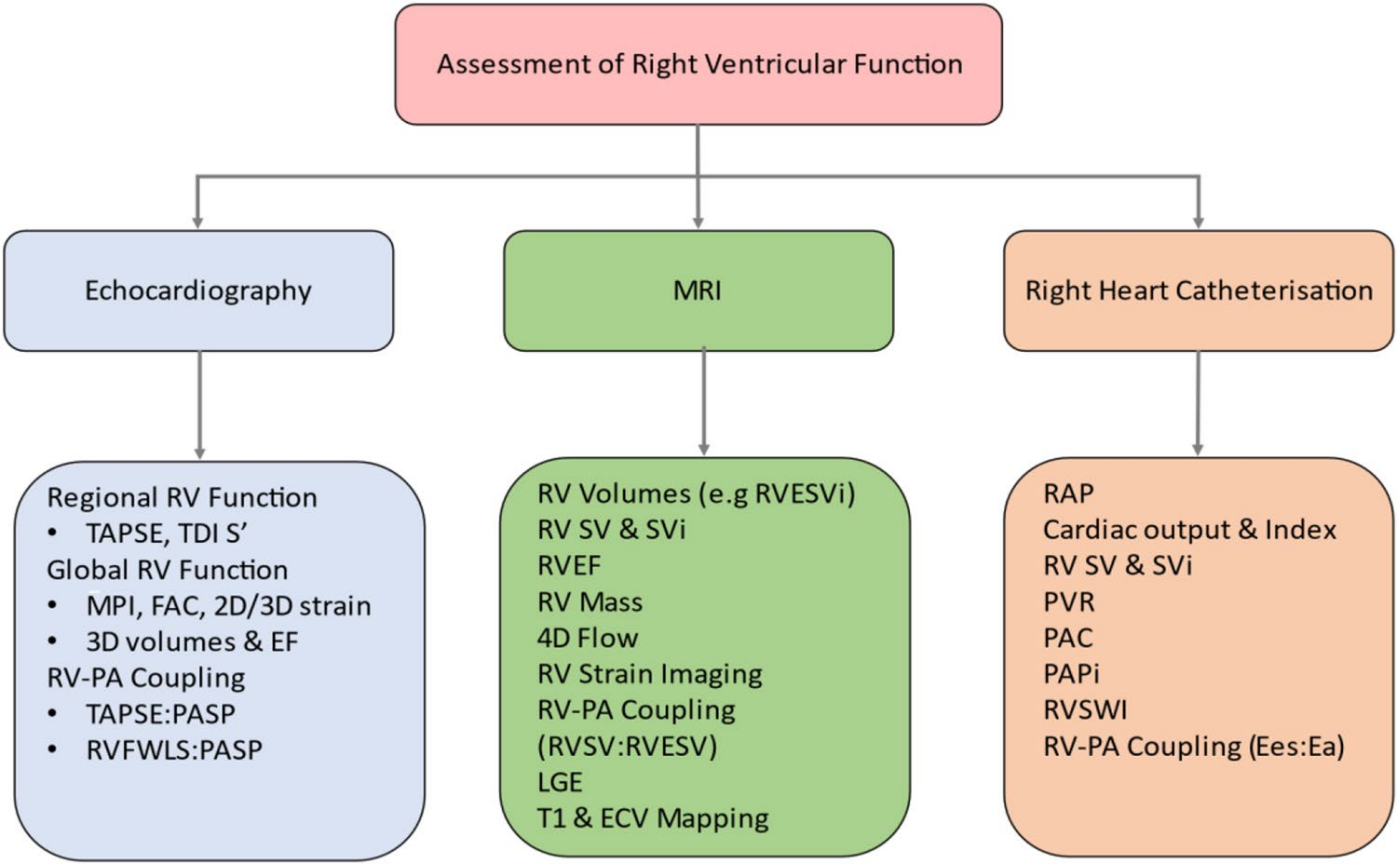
Modalities and parameters used for the assessment of right ventricular function. MRI, magnetic resonance imaging; RV, right ventricular; TAPSE, tricuspid annular plane systolic excursion; TDI S′, right ventricular systolic wave on Tissue Doppler Imaging; MPI, myocardial performance index; FAC, fractional area change; EF, ejection fraction; PA, pulmonary artery; PASP, pulmonary arterial systolic pressure; RVFWLS, right ventricular free wall longitudinal strain; RVESVi, right ventricular end-systolic volume index; SV, stroke volume; SVi, stroke volume index; RVSV, right ventricular stroke volume; RVESV, right ventricular end-systolic volume; LGE, late gadolinium enhancement; ECV, extracellular volume; RAP, right atrial pressure, PVR, pulmonary vascular resistance; PAC, pulmonary arterial compliance; PAPi, pulmonary arterial pulsatility index; RWSVI, right ventricular stroke work index; Ees, end-systolic elastance; Ea, arterial elastance
